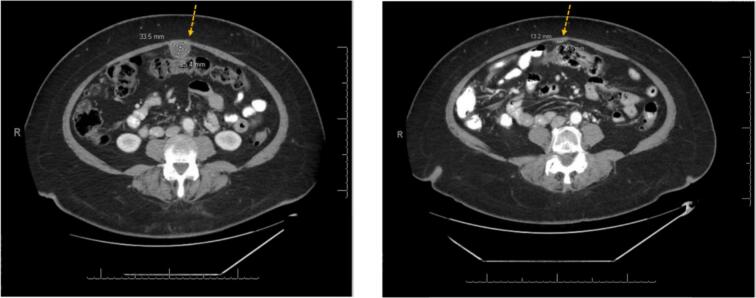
CT imaging demonstrates response to treatment with T-DXd in Case 1. Focal metastatic lesion decreases in size from 33.5 mm x 25.4 mm (left) to 13.2 mm to 5.5 mm (right) with T-DXd treatment over the course of six months.
